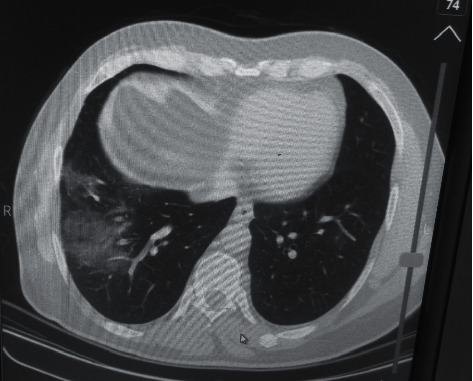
Lung CT scan of the patient at previous admission shows moderate involvement of the patient's lungs.
